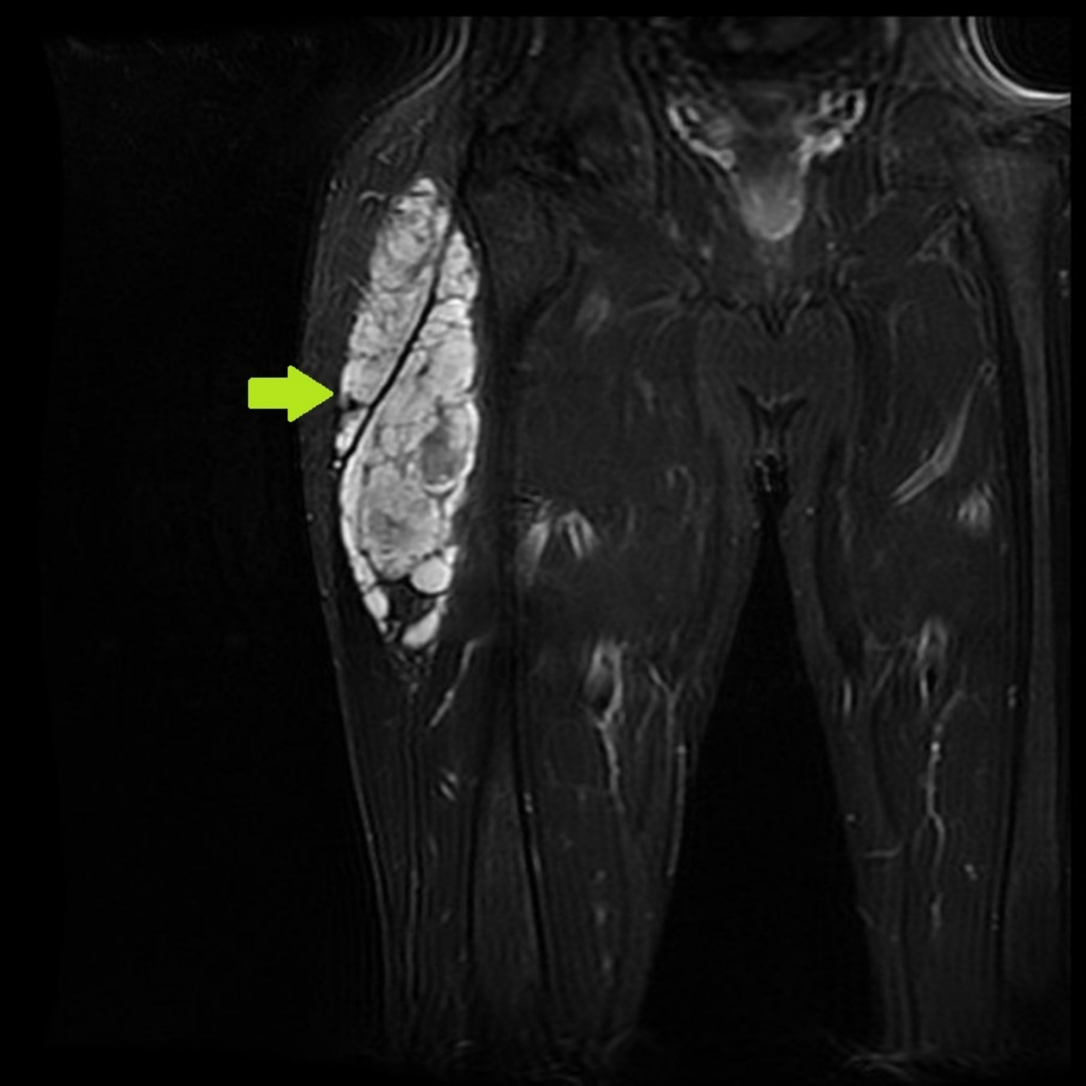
Magnetic resonance imaging (MRI) showing right gluteal extraskeletal myxoid chondrosarcoma.
